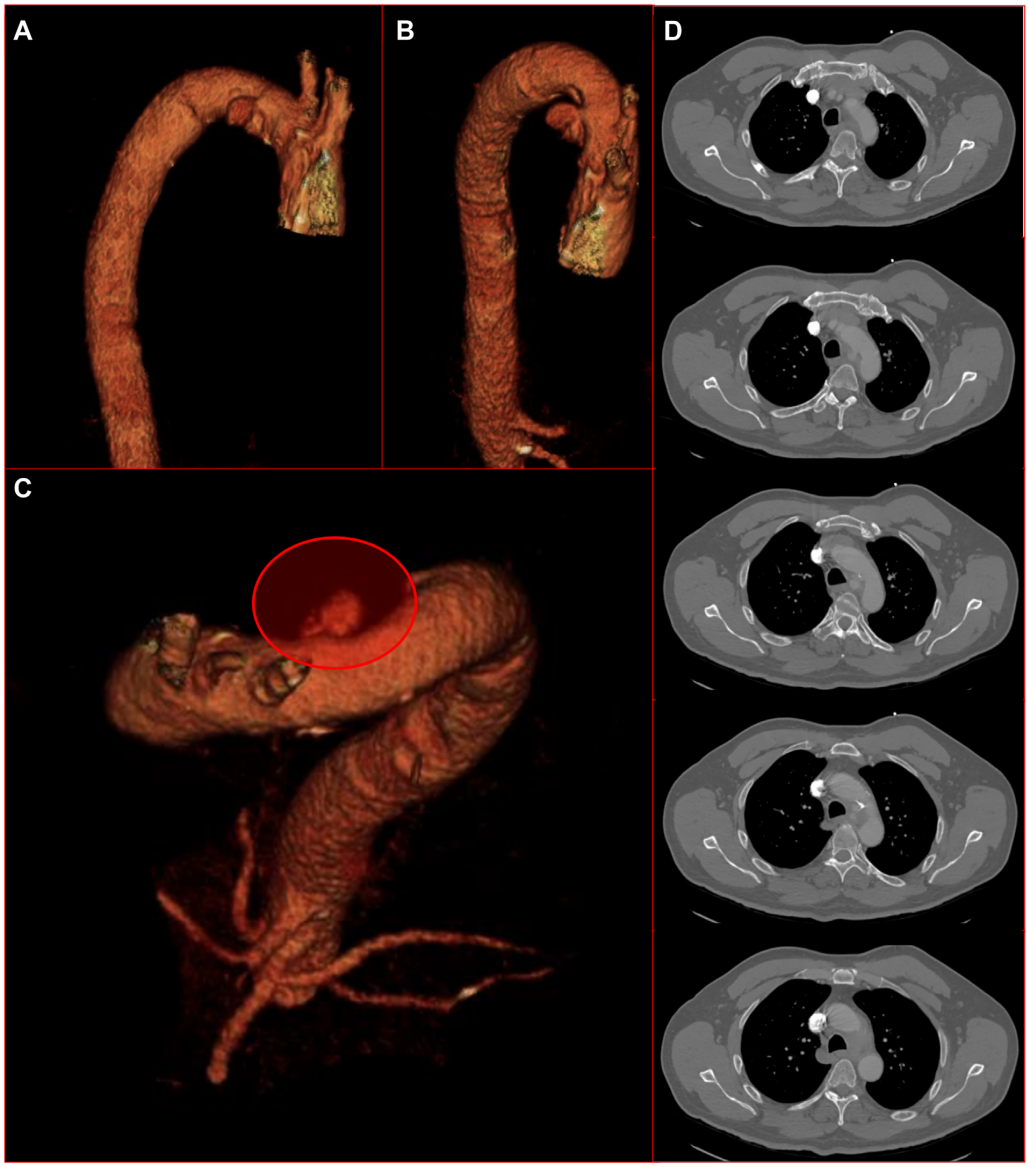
Index diagnostic computed tomography (CT) angiogram revealing posteromedial penetrating aortic ulcer (PAU) vs pseudoaneurysm. Three-dimensional reconstructions of the preoperative CT angiogram. (A) Posterior view (right anterior oblique [RAO], 100; cranial [CRA], 10). (B) Posterior view (RAO, 70; CRA 60). (C) Superior (left anterior oblique [LAO], 50; CRA, 70). (D) Axial CT angiography series.
